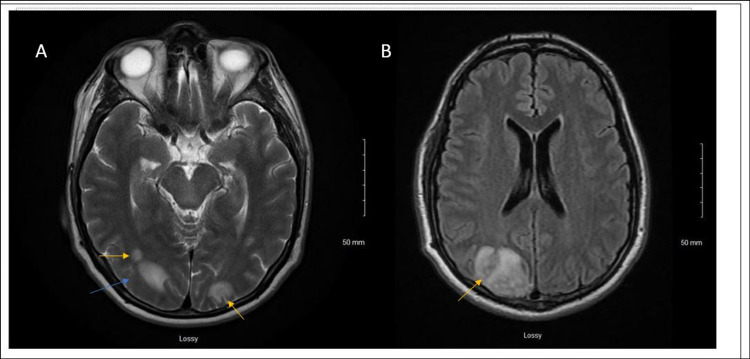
MRI of the brain with multiple brain abscesses secondary to septic emboli from aortic valve endocarditis (yellow arrows in A showing occipital emboli, blue arrow in A concerning for occipital abscess, yellow arrow in B showing a large occipital abscess). MRI: magnetic resonance imaging
